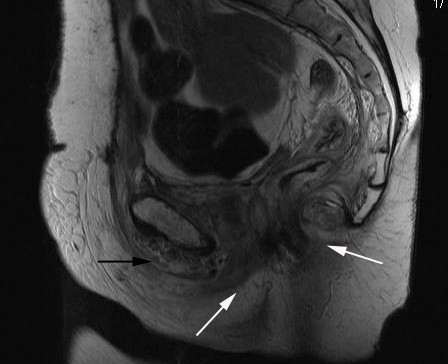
T1-weighted MRI of the pelvis (sagittal view) exhibiting gross oedema of the urethra, vagina, and rectum (white arrows) with an air-fluid level within pubic symphsis extending into peritoneal retropubic space superior to the urethra (black arrow).
